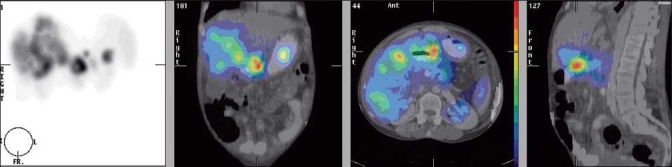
Fusion image of SPECT and CT. From left to right: SPECT MIP view, coronal view, transversal view and sagittal view. Positive uptake is located in liver metastases (diffuse uptake to the right), pancreas (high uptake in centre and in sagittal view) and stomach (left in coronal view)
